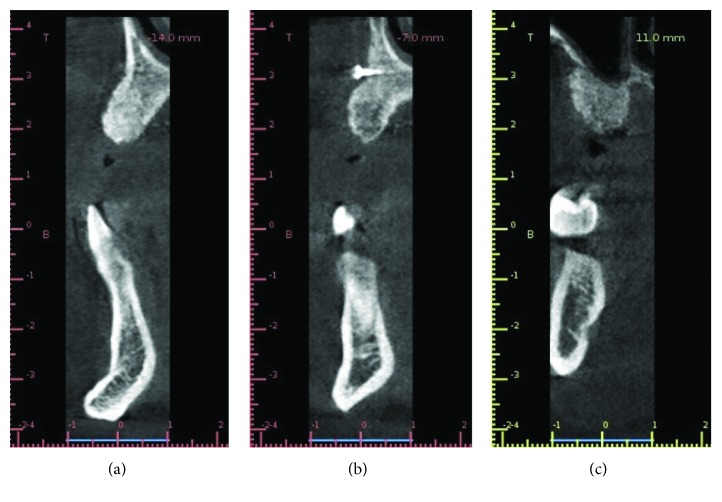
CBCT after healing during 6 months.
